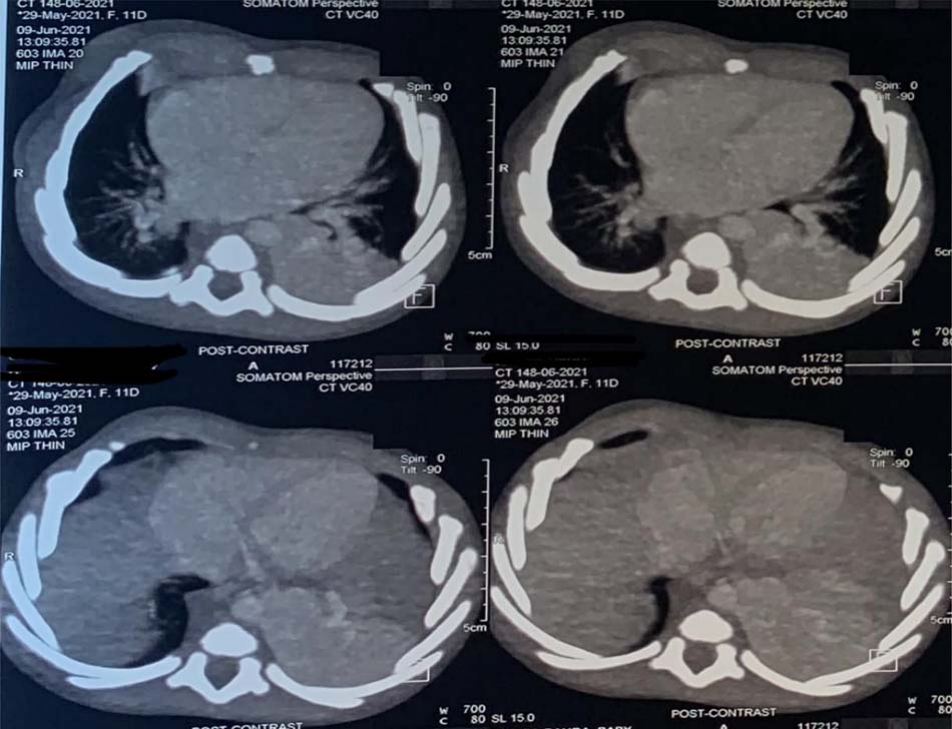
CT scan images depicting intralobar pulmonary sequestration of the left lower lung lobe.
